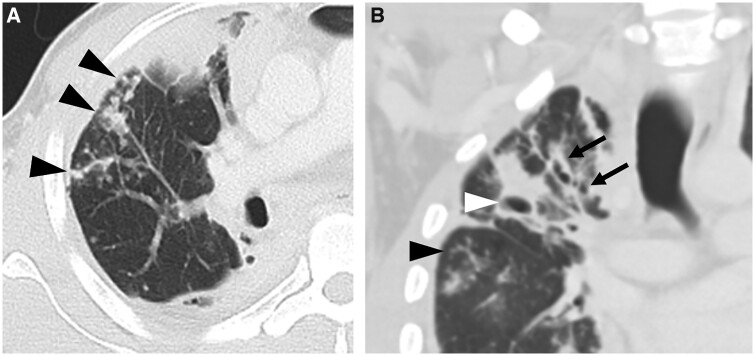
Postprimary TB with tree-in-bud opacities in a 30-year-old man. (A) Axial CT image shows tree-in-bud appearance (black arrowheads) seen as multiple small, centrilobular nodules connected to linear branching opacities. (B) Coronal CT image shows reticulonodular infiltration, cavitary lesion (white arrowhead) and traction bronchiectasis with wall thickening (arrows). Tree-in-bud opacities are also seen (black arrowhead).
